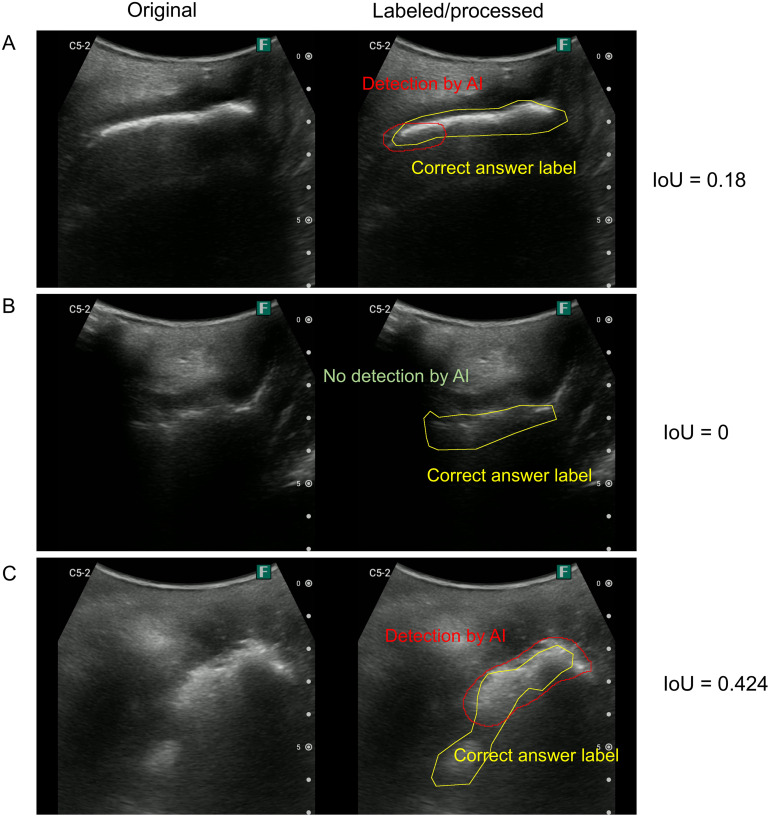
Examples of ultrasound images determined as false negatives. (Left) Original image. (Right) Areas labeled as correct by the sonographer (yellow) and detected by AI (red). (A) IoU = 0.18, (B) IoU = 0, (C) IoU = 0.424, and IoU < 0.5, resulting in a false negative decision. AI, artificial intelligence; IoU, intersection over union.
